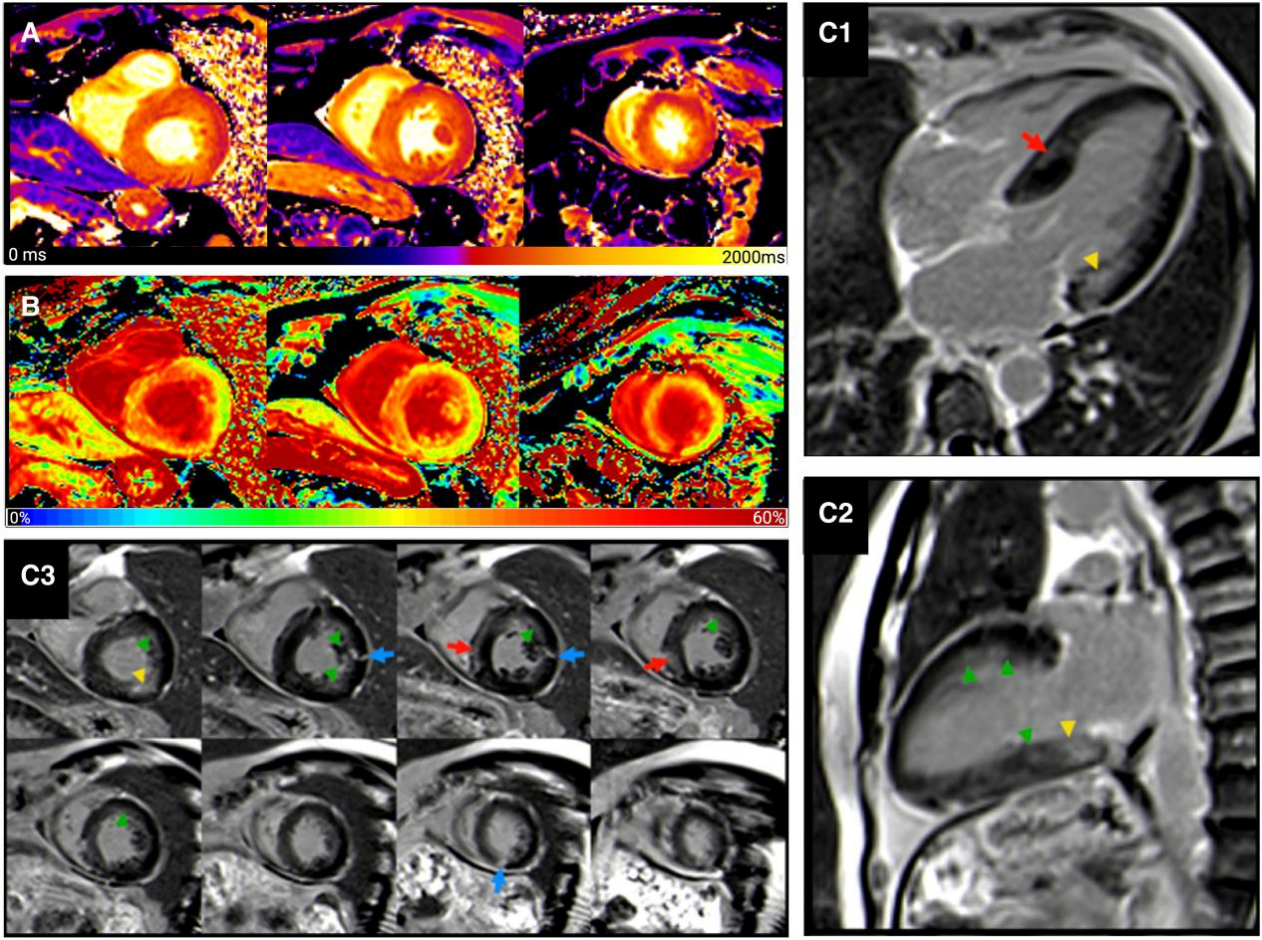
Cardiac magnetic resonance imaging. Native T1 maps (*A*) and extracellular volume maps (*B*) in a basal, mid-ventricular, and apical slice. Mean native myocardial T1 time per slice from base to apex was 1328, 1313, and 1285 ms (*z*-score 2.8, 2.4, and 1.6), and corresponding extracellular volume was 36, 34, and 35%. Late gadolinium enhancement (fast low-angle shot, phase-sensitive inversion-recovery) in a four-chamber view (*C*1), two-chamber view (*C*2), and short-axis stack from basal to apical (*C*3) showed indistinct subendocardial late gadolinium enhancement in the basal segments (green arrowheads) with hazy late gadolinium enhancement especially in the basal inferolateral segment (yellow arrowheads), patchy mid-myocardial septal late gadolinium enhancement (red arrows), and few small almost transmural foci of late gadolinium enhancement in the lateral and inferior wall (blue arrows). Additionally, the right ventricle presented normal in size and function without apparent wall thickening or detectable late gadolinium enhancement, while late gadolinium enhancement of the atrial walls could have been suspected in *C*1.
